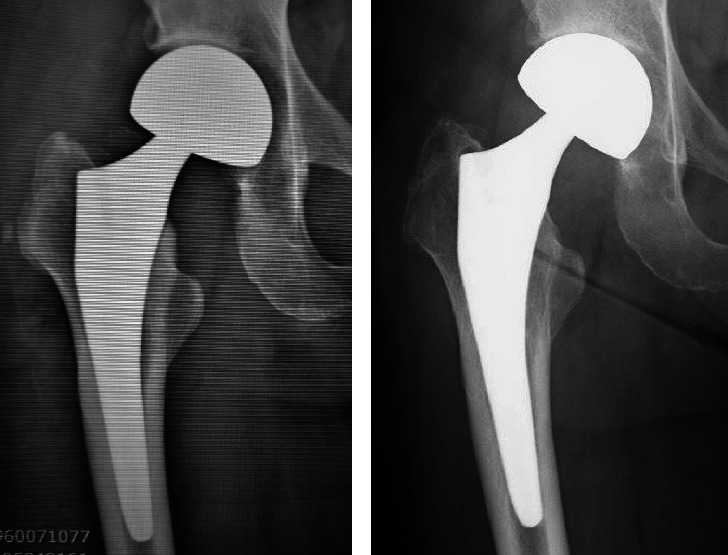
Plain radiograph of the right hip. (a) After initial surgery with BHA. (b) Two years after initial BHA.
